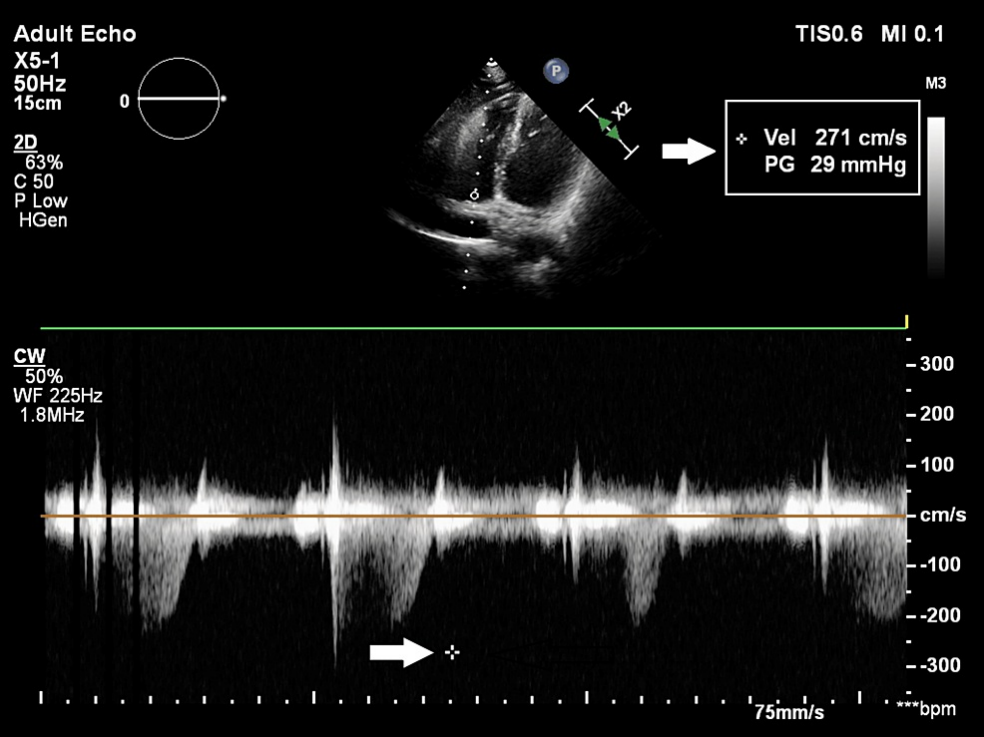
2D echo showing mild pulmonary hypertension The pressure gradient across the tricuspid valve shows 29 mmHg (white arrow). 5 mmHg pressures were added to the tricuspid valve pressure gradient since the inferior vena cava was normal. Right ventricular systolic pressure was derived as 34 mmHg, which indicated mild pulmonary hypertension. 2D echo: two-dimensional echocardiography
